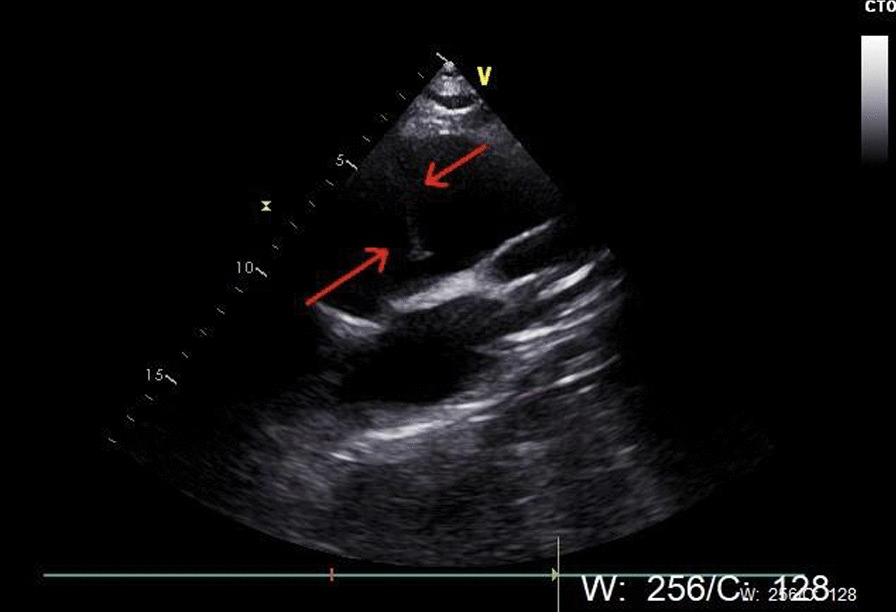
A flap floating in the aorta in the long axis of the aorta on transthoracic echocardiography
